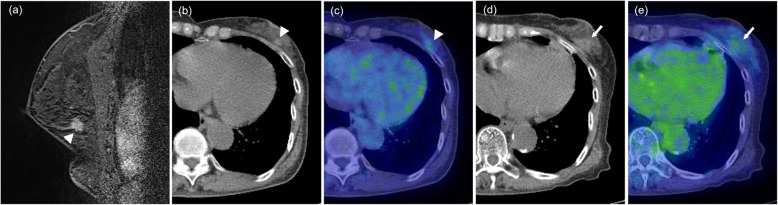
Non-fatty mass. A 73-year-old woman with invasive ductal carcinoma. Breast MRI before cryoablation (a, arrowhead) showing an irregularly shaped nodule of diameter 11 mm in the outer-lower quadrant of the left breast. The pre-cryoablation CT portion (b, arrowhead) and fusion image (c, arrowhead) of PET/CT show a solid nodule with abnormal FDG uptake (SUVmax, 1.78). The CT portion (d, arrow) and (e, arrow) fusion image of PET/CT that was obtained 11 months after cryoablation shows a non-fatty mass and subtle FDG uptake in the treated area (SUVmax, 1.86)
